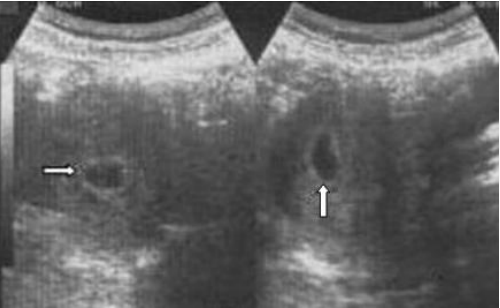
Ultrasonographic image of the case, monochorionic triamniotic triplet pregnancy, is demonstrating single chorionic cavity at 6 weeks of gestation (arrows).
